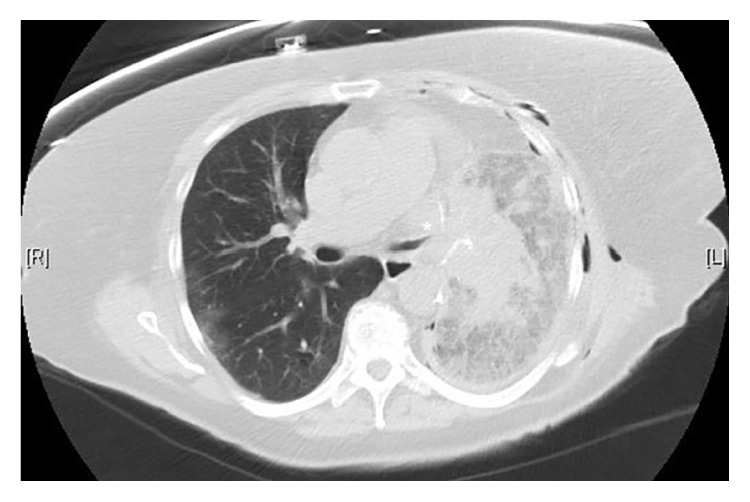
CT of the chest parenchymal view demonstrating left lobar consolidation with ground glass attenuation and proximal bronchial tapering (white asterisk).
